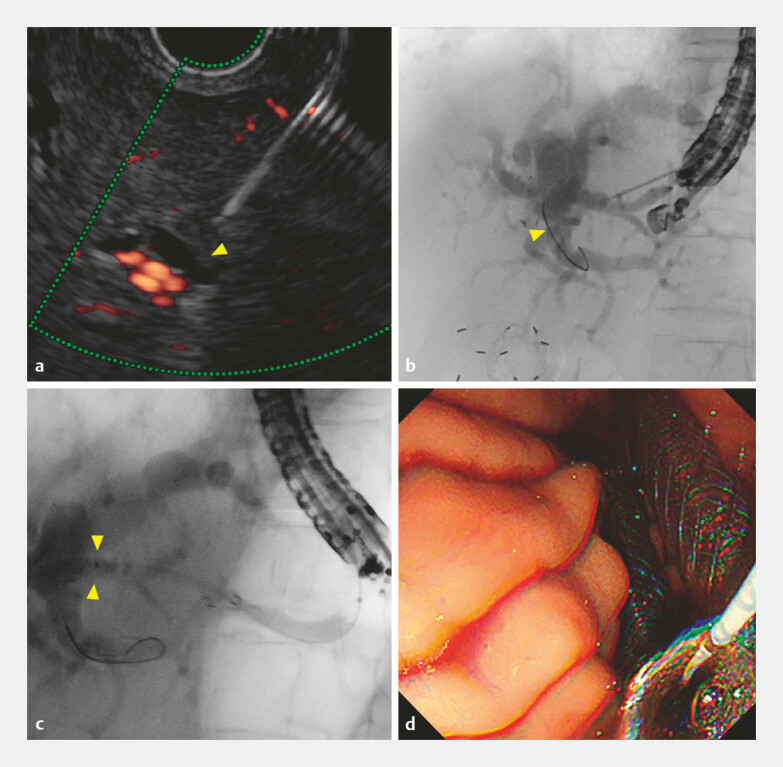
Images during the second successful endoscopic ultrasound-guided hepaticogastrostomy.
